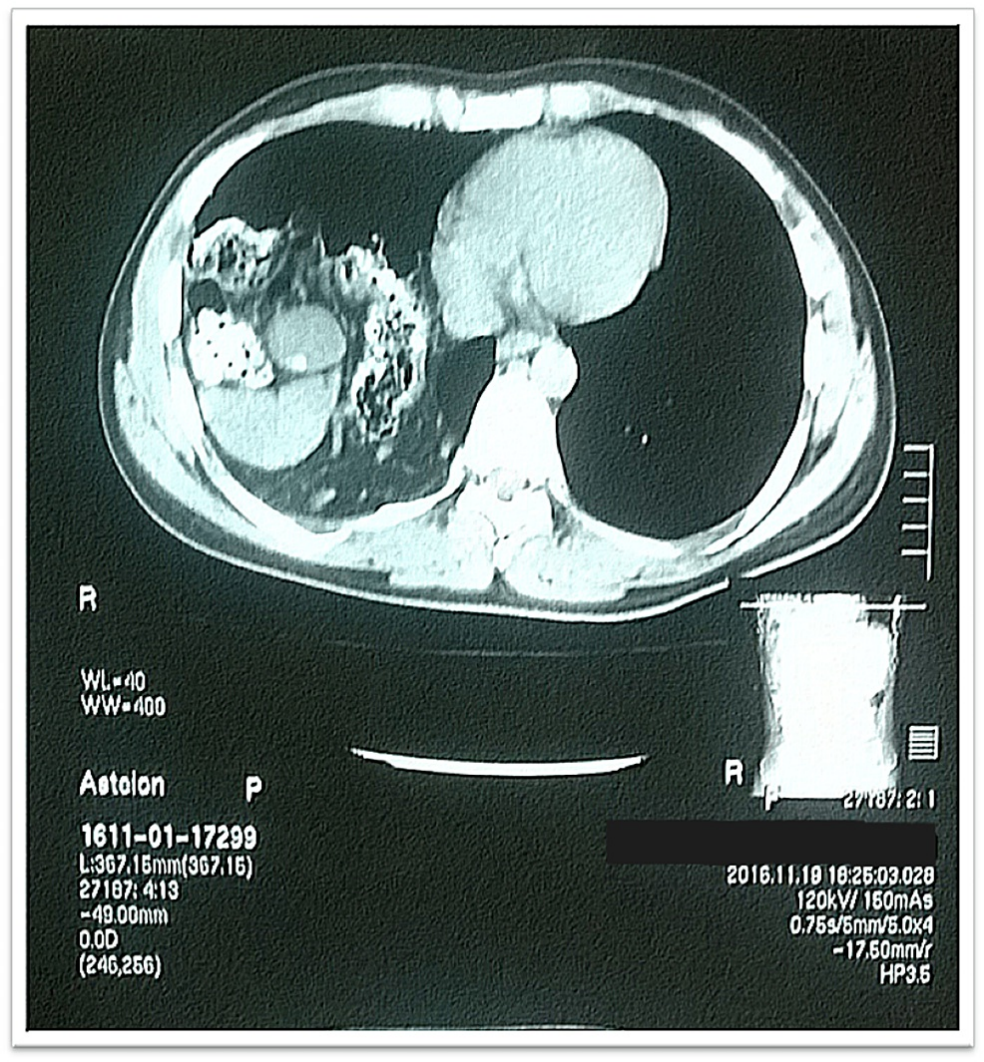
CT image showing herniated contents in the right hemithorax with a calculus in the gallbladder - Axial view CT - Computed Tomography
